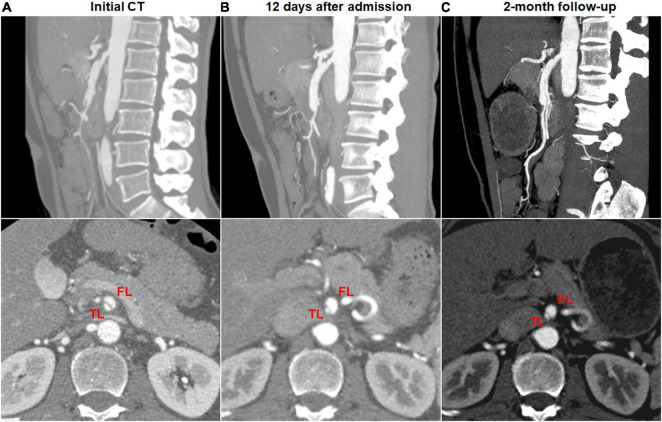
Morphologic progression of type II spontaneous isolated superior mesenteric artery dissection on follow-up computed tomography (CT) angiography. (A) Initial CT angiography showed the patent “cul-de-sac” shaped false lumen without re-entry in a 48-year-old man. (B) Twelve days later, blind-ending false lumen was prolonged and compressed the true lumen despite symptomatic relief. (C) Two months later, the false lumen was further enlarged, and true lumen remained severely stenosed.
